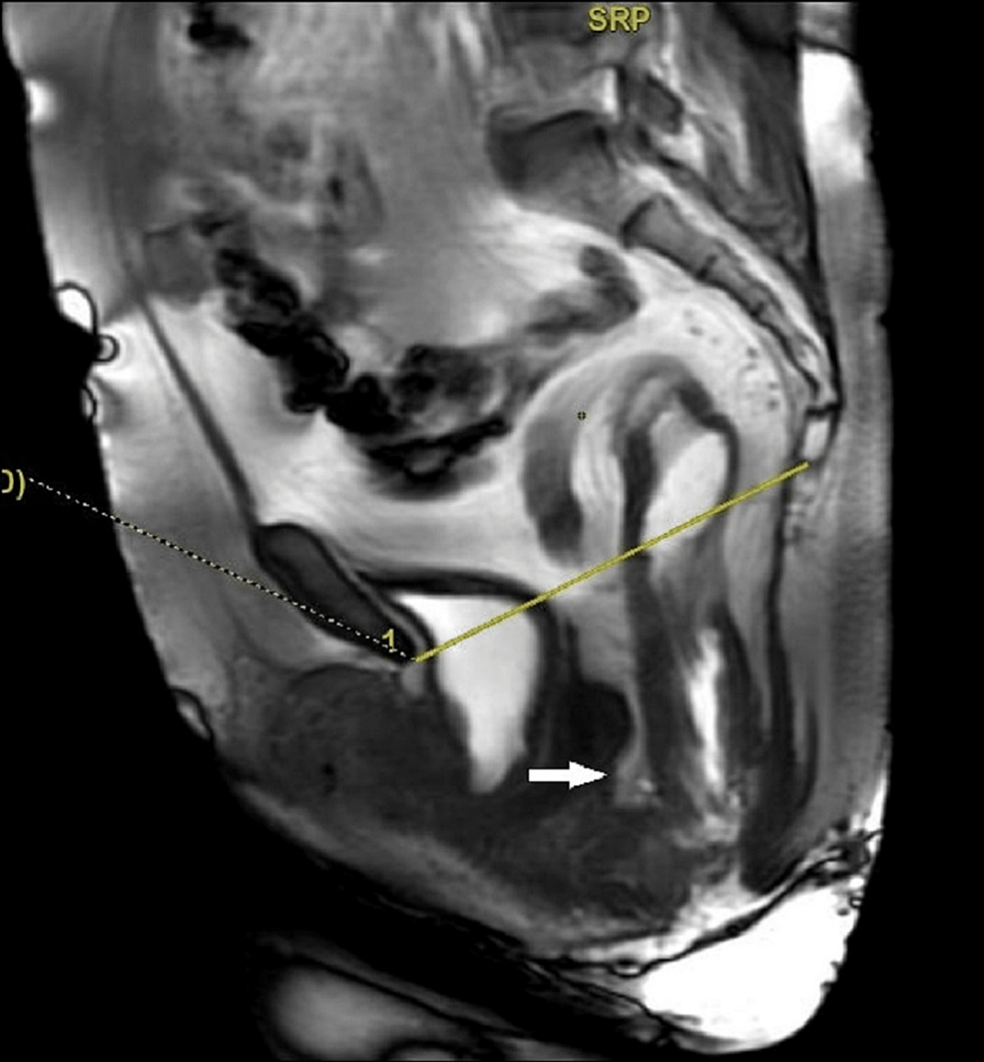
A 53-year-female, post-hysterectomy, with pain during defecation. Midline sagittal dynamic FIESTA MRI image at the end of the defecation phase reveals peritoneocele (white arrow). Also, note the intra-anal rectal mucosa intussusception. FIESTA: fast imaging employing steady-state acquisition.
